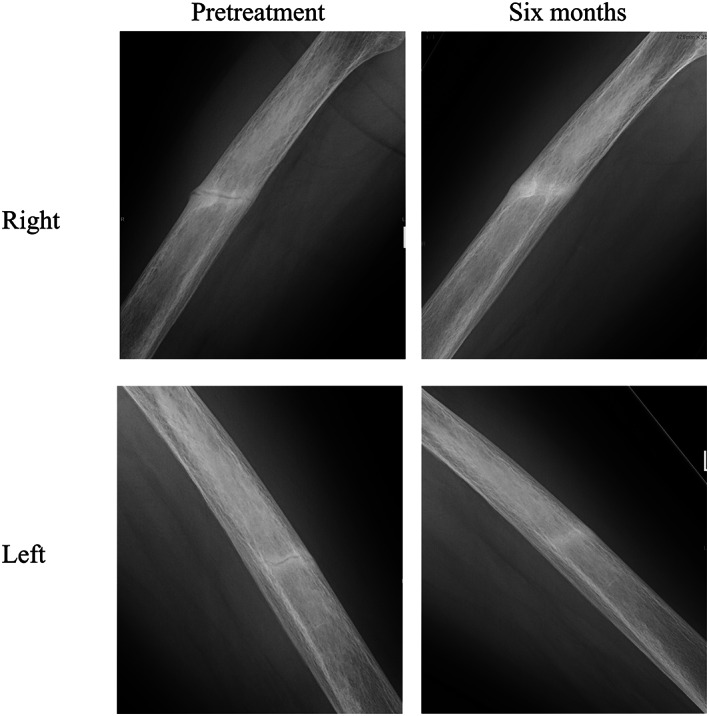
Radiographic improvement of the pseudofractures observed in bilateral femoral diaphyses, 6 months after the initiation of asfotase alfa.
